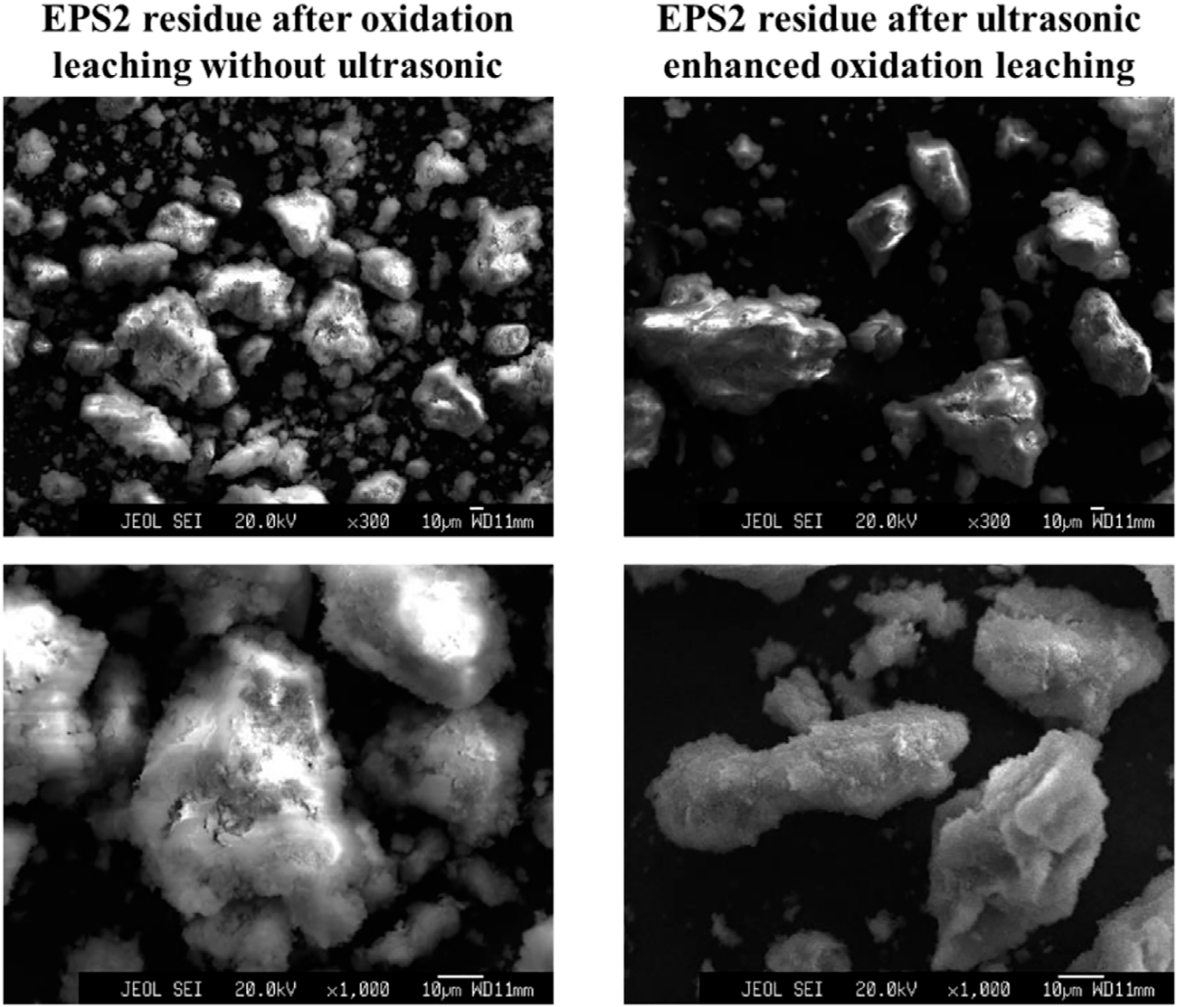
Particle morphology of complex sludge (EPS2) after oxidation leaching with and without ultrasonic treatment.
